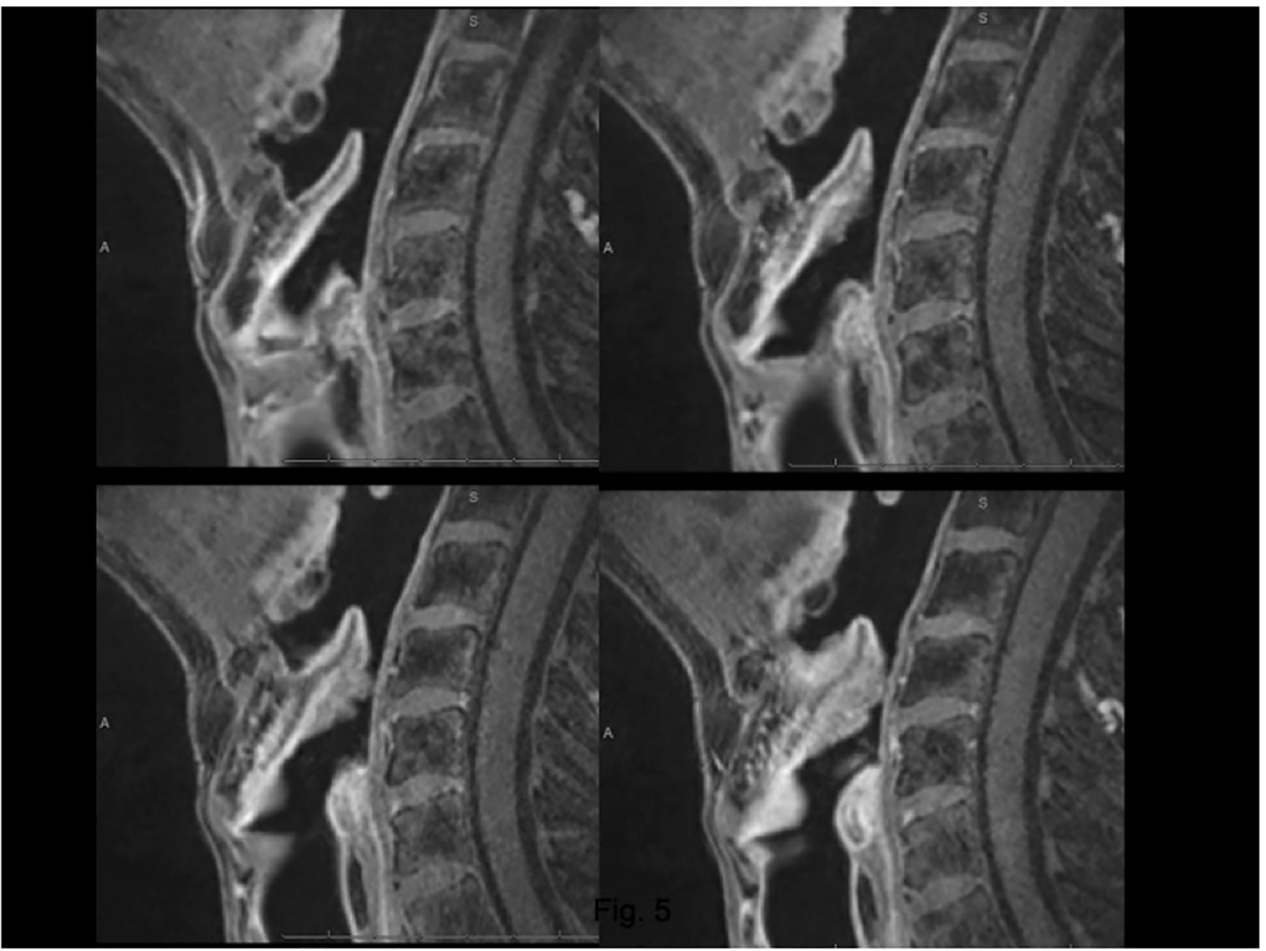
MRI, T1 fat-sat sequence (VIBE) on sagittal plane. Lesion arising from the epiglottis, which infiltrates both its supra- and infrahyoid portions, up to the petiole. Effacement of the posterior part of the median pre-epiglottic space.
